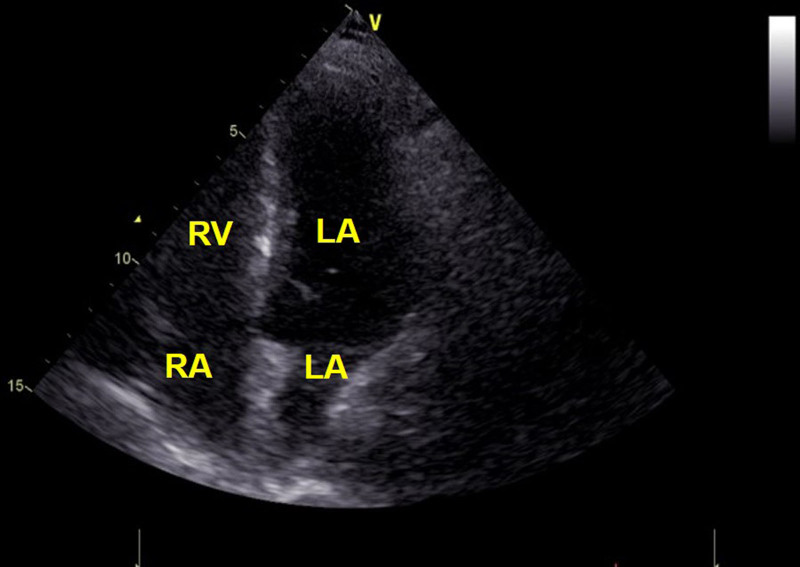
Preoperative transthoracic echocardiography (TTE) in the apical 4-chamber view demonstrates normal morphology of the right atrium and right ventricle, with no evidence of thrombus or intracardiac mass. LA = left atrium, LV = left ventricle, RA = right atrium, RV = right ventricle.
